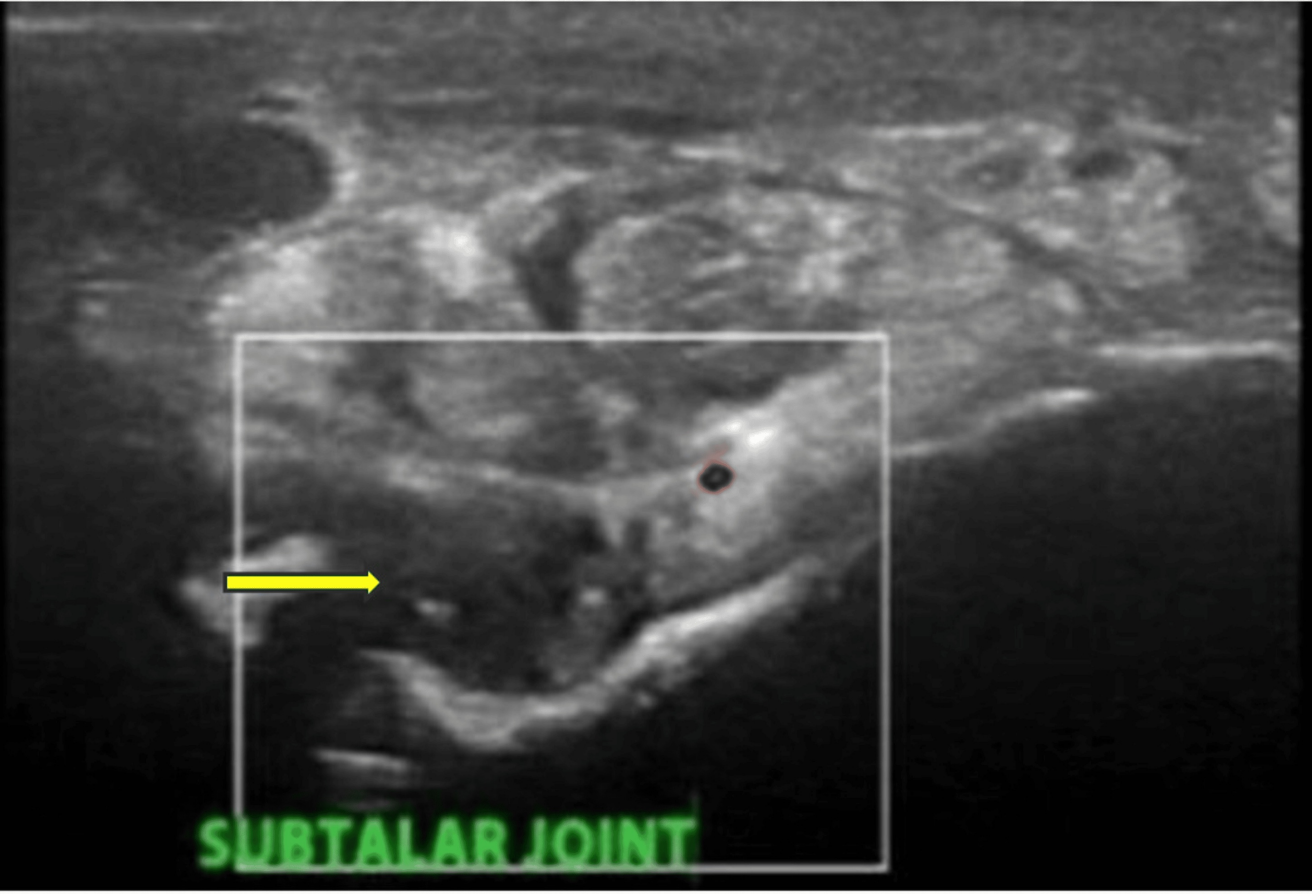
Ultrasonography (USG) of subtalar joint showing synovial hypertrophy with grade 1 Doppler The yellow arrow indicates a hypoechoic area in the subtalar joint with grade 1 Doppler.
